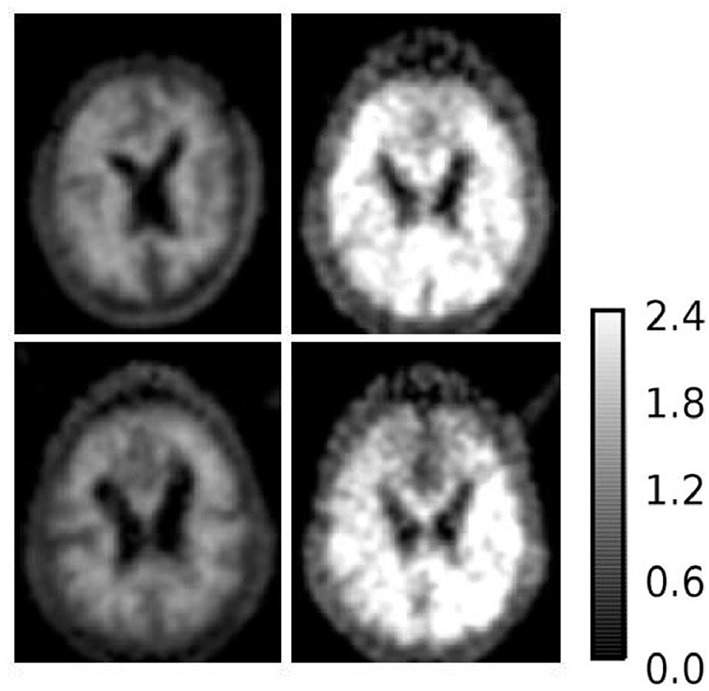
SUVR images of example AD subjects (1) upper left: AD_038 (SUVR = 1.1207); (2) upper right: AD_001 (SUVR = 2.0235); (3) lower left: AD_040 (SUVR = 1.1699); (4) lower right: AD_005 (SUVR = 1.5531). AD_038 and AD_040 were misclassified as HC subjects by SUVR and DVR. AD_001 and AD_005 were correctly classified AD subjects by all three measures.
